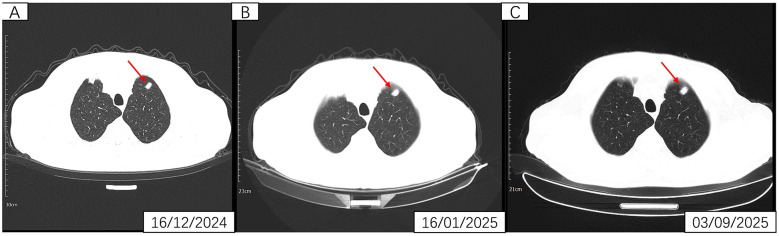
(A-C) Postoperative follow-up chest CT shows stable lung metastases with no significant change in size, taking a larger nodule as an example.
